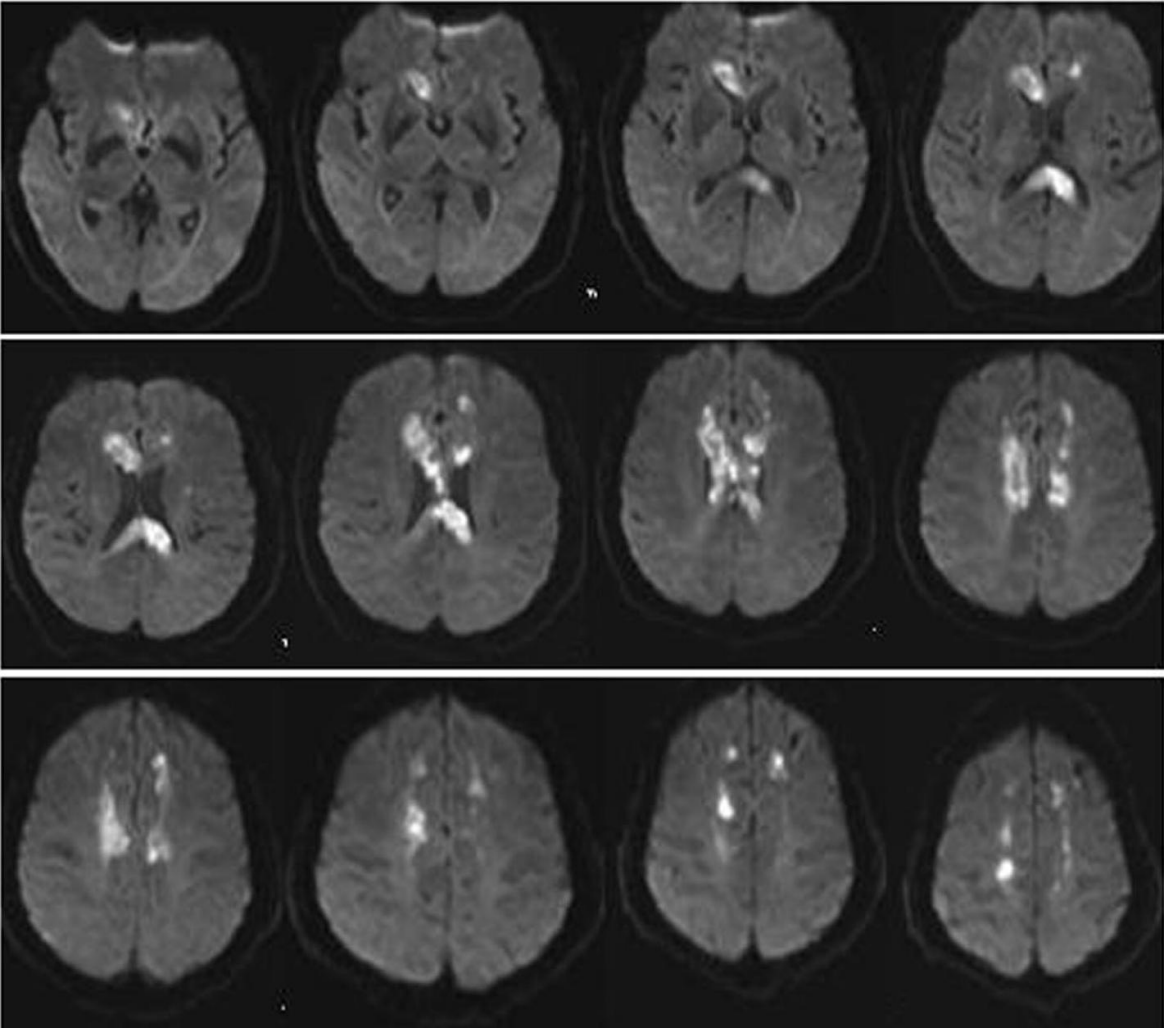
Axial DWI showed high signal intensity in all the slices of the lesions of the corpus callosum.
